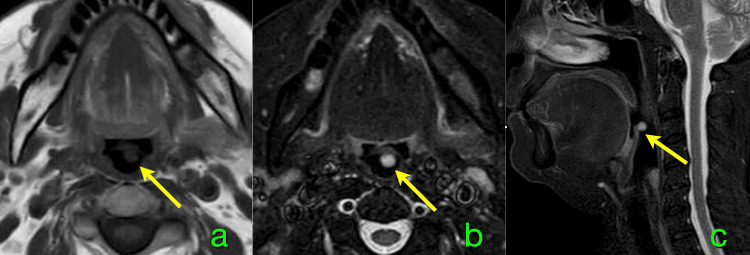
MRI images of this patient This lesion (arrow) shows low-signal intensity on axial T1-weighted image (a), high-signal intensity on axial (b) and sagittal (c) short tau inversion recovery images. This lesion does not infiltrate the epiglottis and is limited to the superficial layer (c) MRI: magnetic resonance imaging
